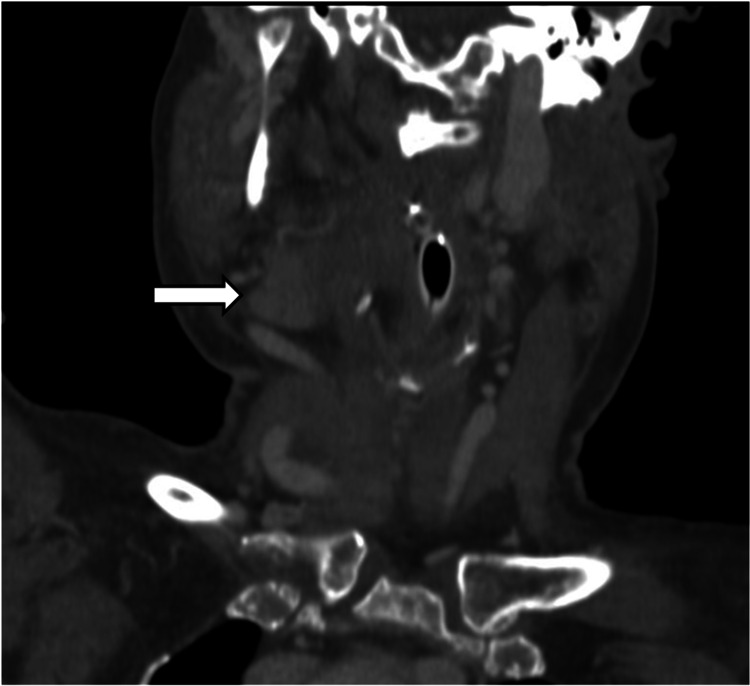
CT showing right cervical hematoma (arrow) with airway compression, coronal view.
